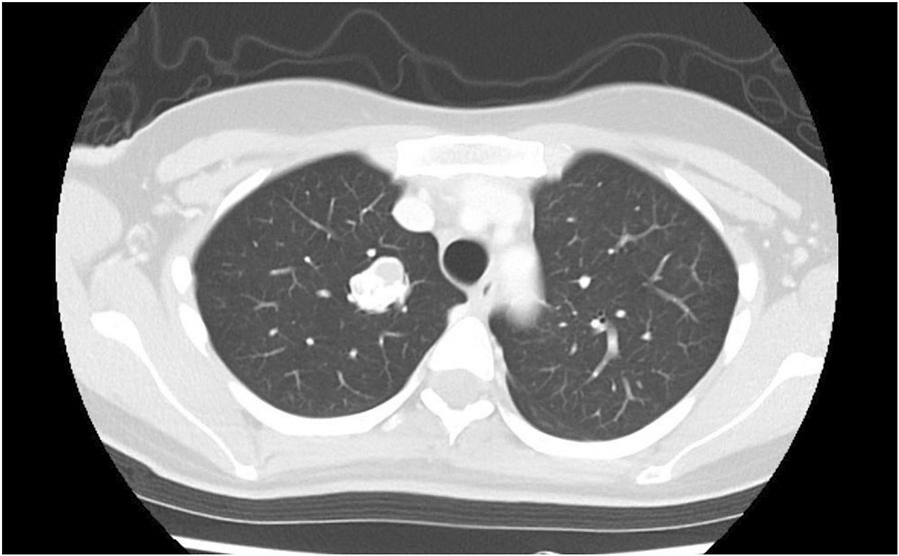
Chest plain CT; there was a nodule with coarse calcifications in the upper lobe of the right lung
